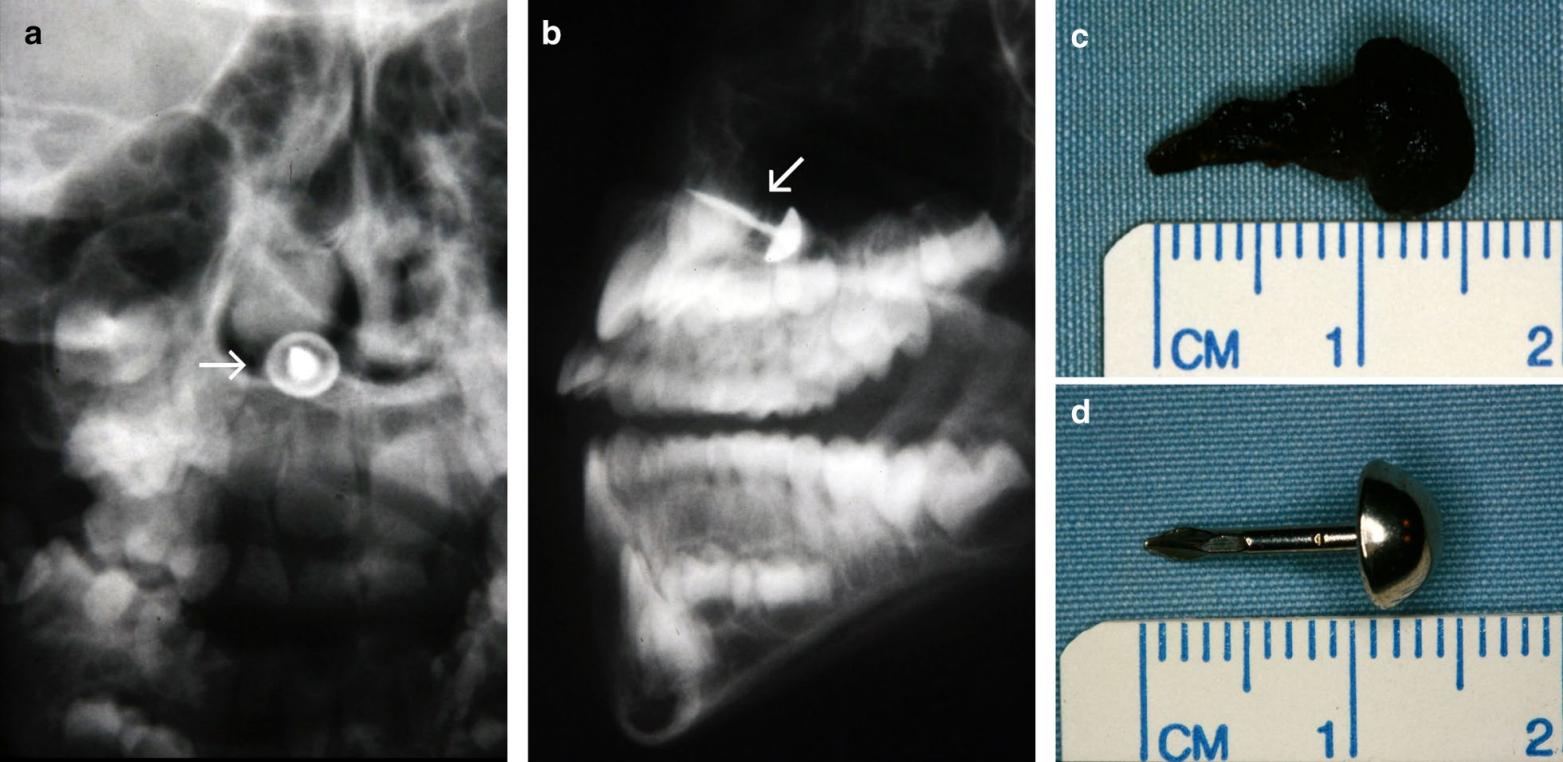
Carpet nail. Anteroposterior (a) and lateral (b) radiographs displaying a radiopaque object (arrow) in projection over the lower right nasal meatus. Photographs of a dislocated carpet nail after removal from the lower meatus (c, d). Adherent blood clots can impair the clinical detection of foreign objects
